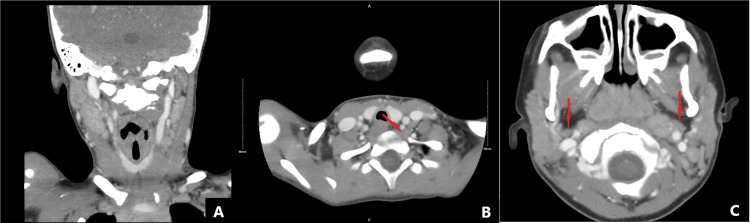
CT scan of the neck with contrast revealing (A) enlarged adenoids, (B) internal jugular lymphadenopathy (arrow), and retropharyngeal lymphadenopathy (arrows) without any masses. CT: computed tomography.
